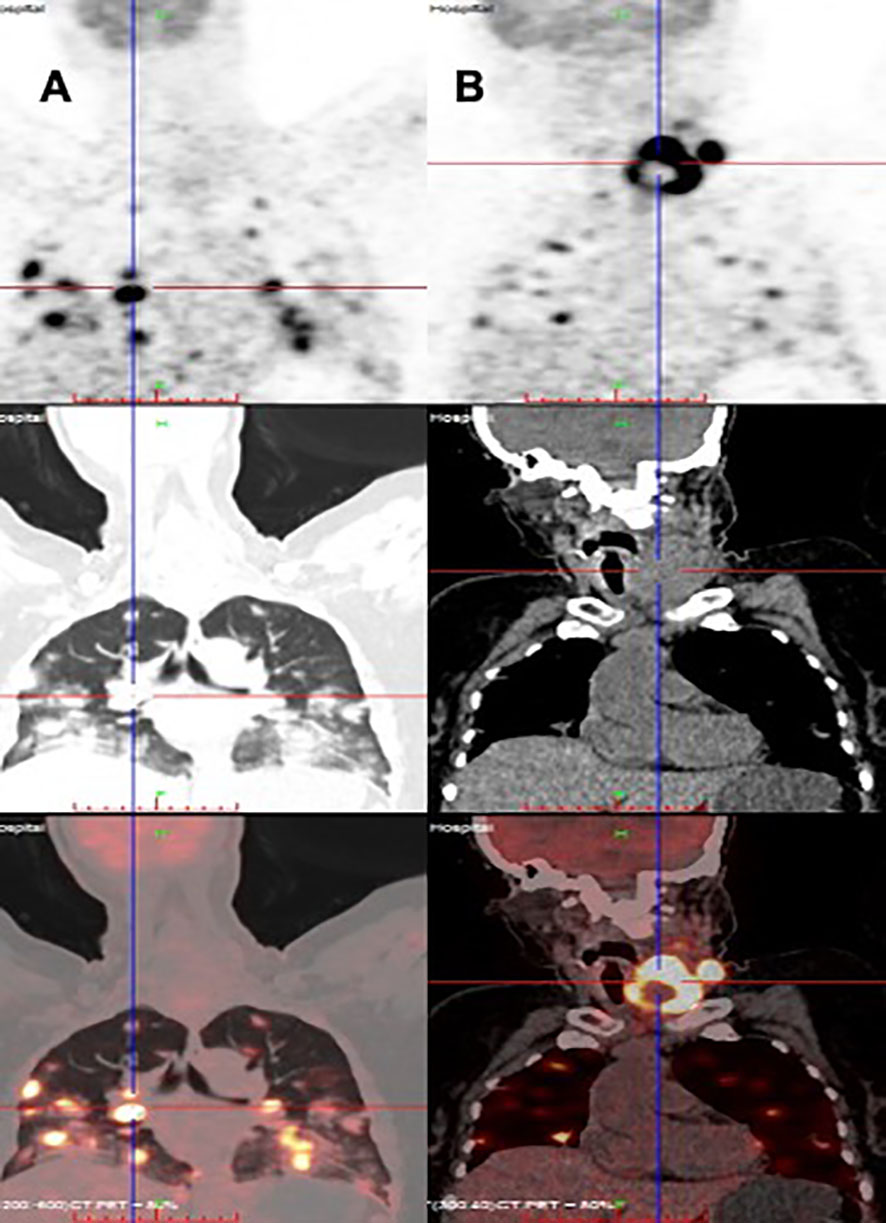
Distant metastasis of PTC in a HIV-infected patient (PET/CT). (A) Multiple lung metastases, (B) papillary thyroid carcinoma.
